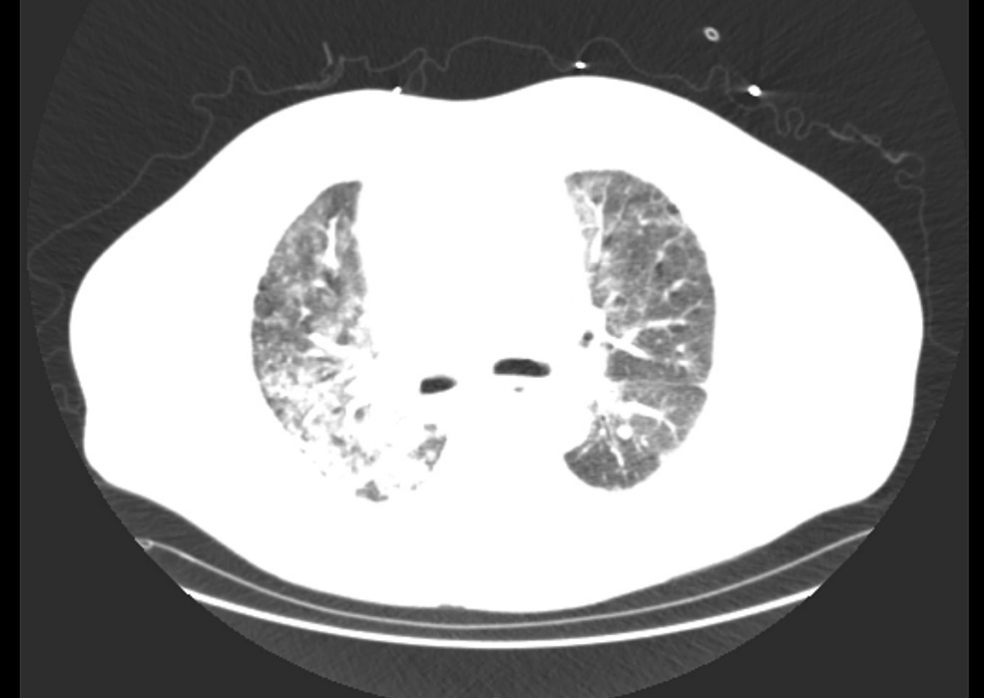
CT chest with contrast-diffuse mosaic attenuation with ground-glass and reticulonodular opacifications on a background of previously visualized fibrotic and bronchiectatic changes with lower lobe predominance and pleural thickening.
